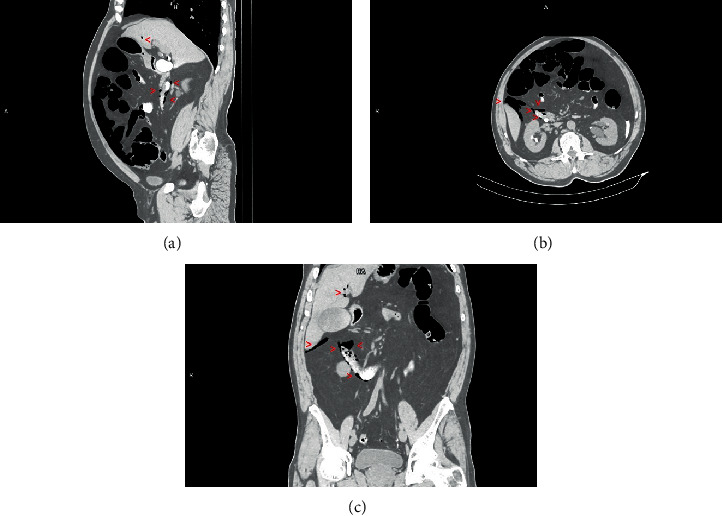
CT—free air around the duodenum in level of the papilla around the liver and visible retroperitoneum around the liver.
